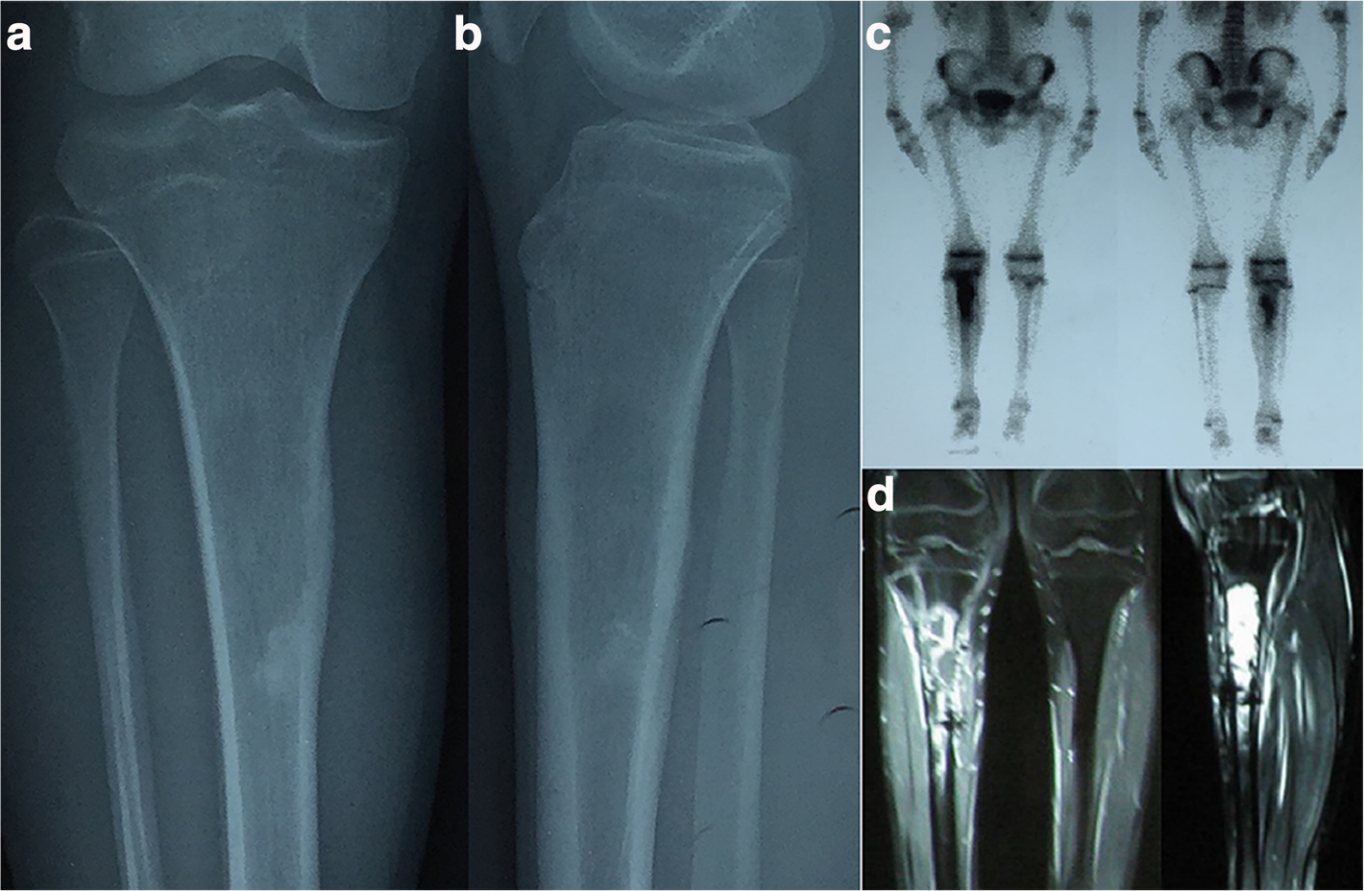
Anteroposterior (a) and lateral (b) radiographs, bone scan (c), and MRI (d) showing metaphyseal osteosarcoma of the right proximal tibia
